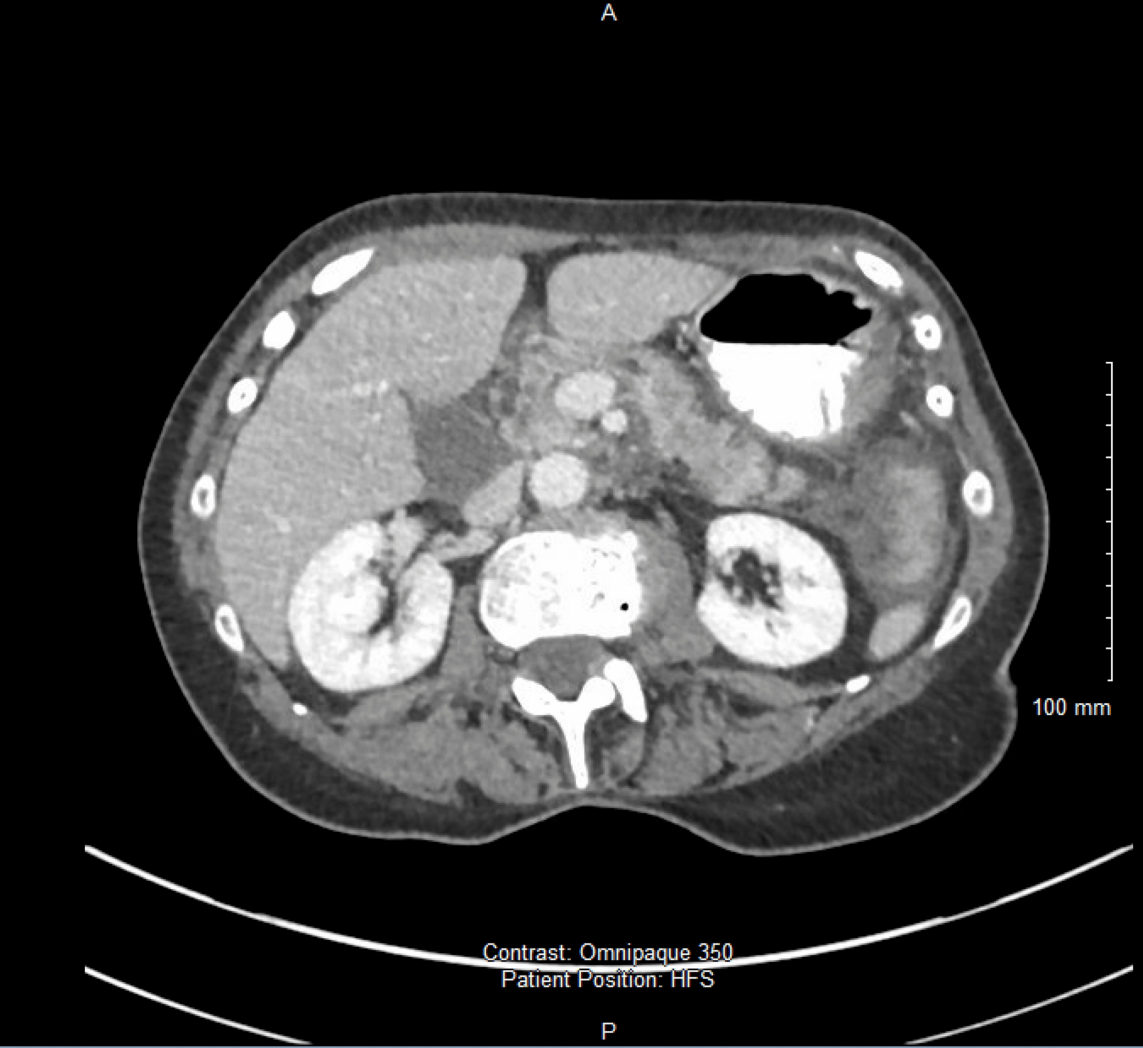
Axial contrast-enhanced CT image of the upper abdomen on follow-up showing resolution of peripancreatic inflammatory changes with normal pancreatic enhancement and clear peripancreatic fat planes, consistent with recovery from acute interstitial pancreatitis
